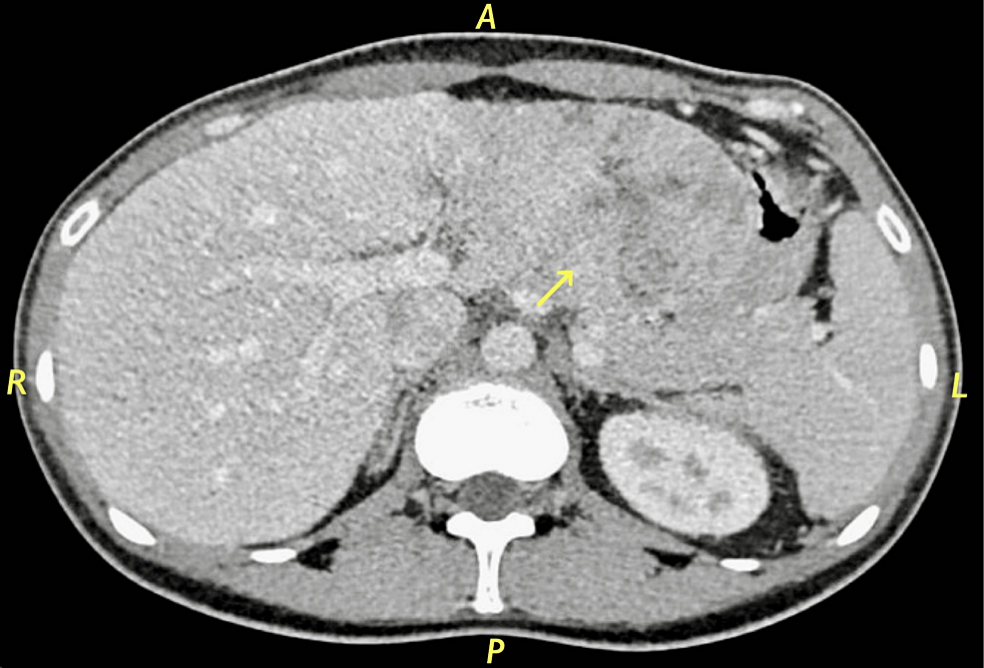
Liver triphasic CT hepatic mass consistent with fibrolamellar carcinoma
